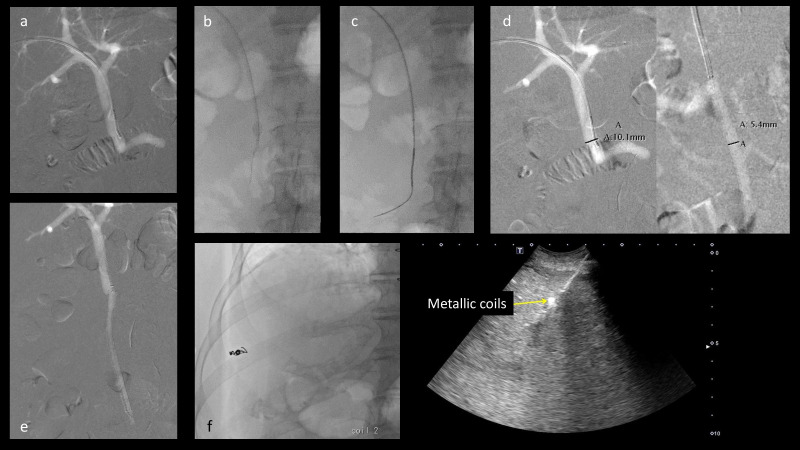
Percutaneous transhepatic portal vein stenting with carbon dioxide (CO_2_) angiography. (a) After a 4-Fr multipurpose pre-shaped catheter was advanced to the main portal vein (PV), angiography revealed that the superior mesenteric vein (SMV) and a portion of the PV were totally occluded. The left gastric vein was visualised. (b) The guidewire and the 4-Fr catheter were advanced approximately 1 cm into the occlusion. (c) After the micro-guidewire was passed through the occlusion, the catheter was advanced into the ileocolic vein. The 4-Fr catheter was advanced over the micro-catheter to reach the ileocolic vein. The 0.035-inch stiff straight wire was then inserted. (d) On CO_2_ angiography, the diameter of the main PV and ileocolic vein was 10.1 and 5.4 mm, respectively. A 6 mm × 10 cm self-expandable metallic stent was deployed from the ileocolic vein to the PV trunk. (e) Stent patency was confirmed with CO_2_ angiography. (f) The puncture route was embolised using two metallic coils under ultrasound guidance.
